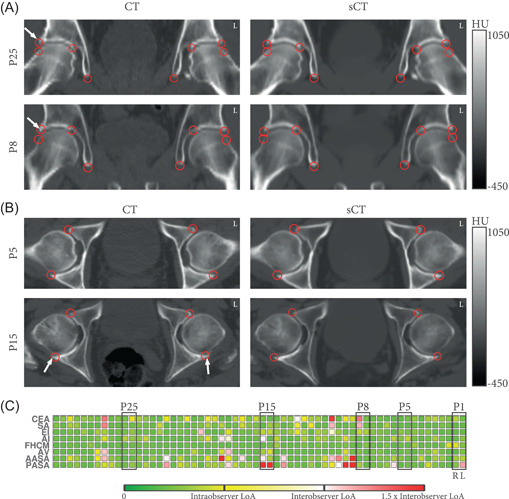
Comparison between CT and sCT. (A and B) Anatomical landmarks (red circles) as annotated by Reader 2 on the CT and sCT for (A) two patients in the coronal plane and (B) two patients in the axial plane. Patients 8, 15, and 25 presented osteophytes (white arrows) around the acetabular rim. (C) Difference between the CT and sCT measurements for each hip joint (*n* = 60). The color coding relates each difference to the intra‐ and interobserver limits of agreement (LoA) of the measurement. For comparison, patients showed in (A) and (B) are highlighted. AASA, anterior acetabular sector angle; AI, acetabular index; AV, acetabular version; CEA, center‐edge angle; CT, computed tomography; EI, extrusion index; FHCM, femoral head center‐to‐midline distance; L, left; PASA, posterior acetabular sector angle; R, right; SA, sharp angle; sCT, synthetic computed tomography [Color figure can be viewed at wileyonlinelibrary.com]
